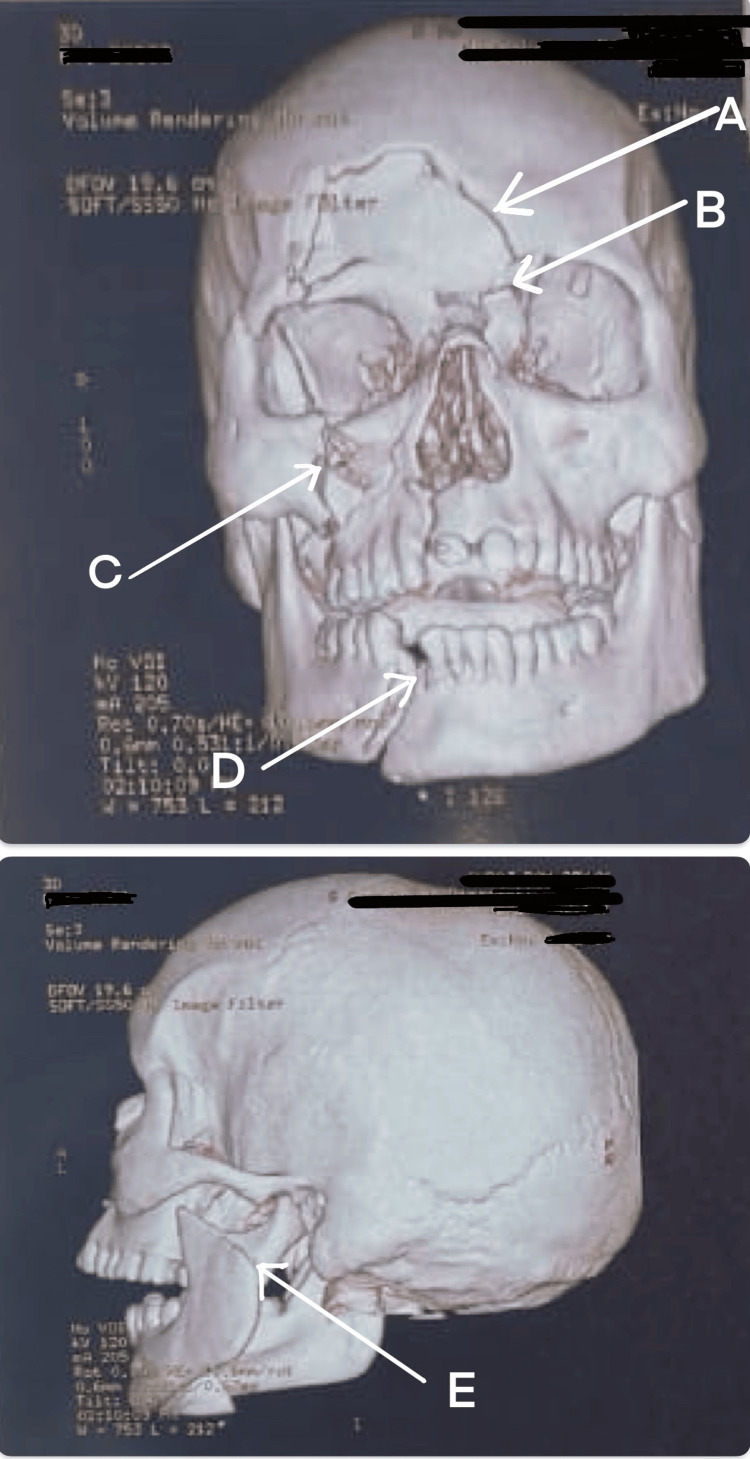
3D CT of the face and skull (Case 2) (A) Fracture of the right frontal bone. (B) Fracture of the left fronto-orbital suture. (C)Fracture of the right zygomaticomaxillary complex (ZMC). (D) Fracture of the right mandibular parasymphysis. (E) Fracture of the left condyle.
